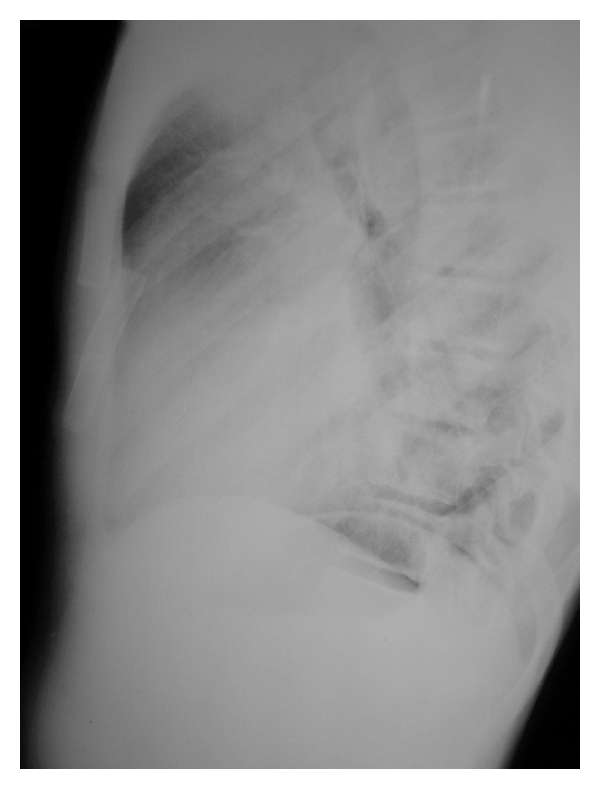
Lateral chest X-ray. Dislocated sternal segments.
